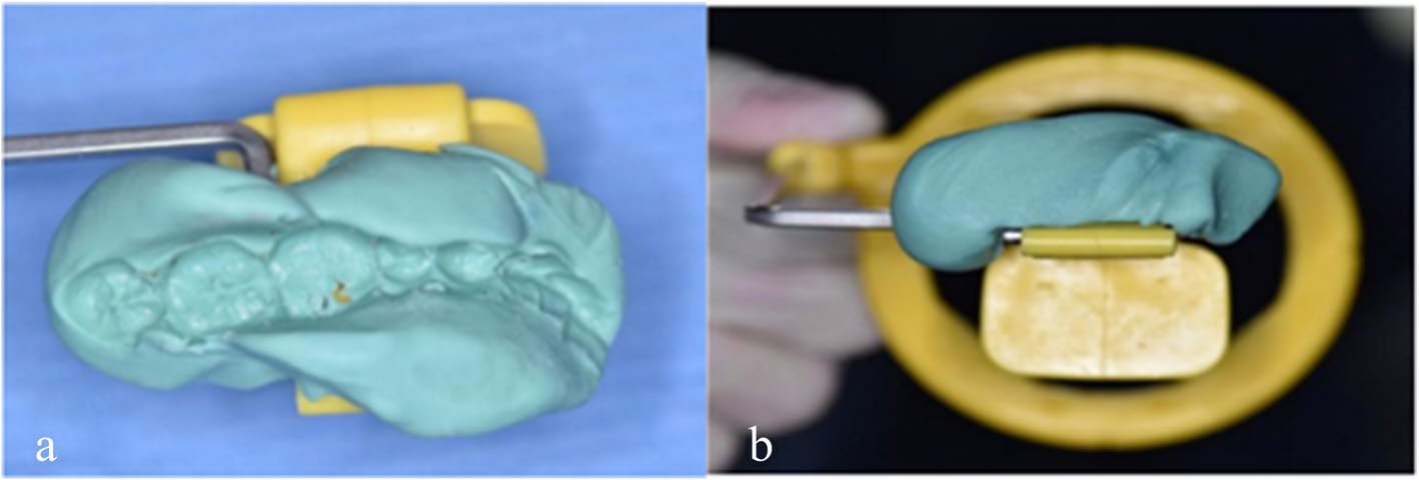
Customized bite registration stent with parallel x-ray holder for a standardized periapical radiograph
